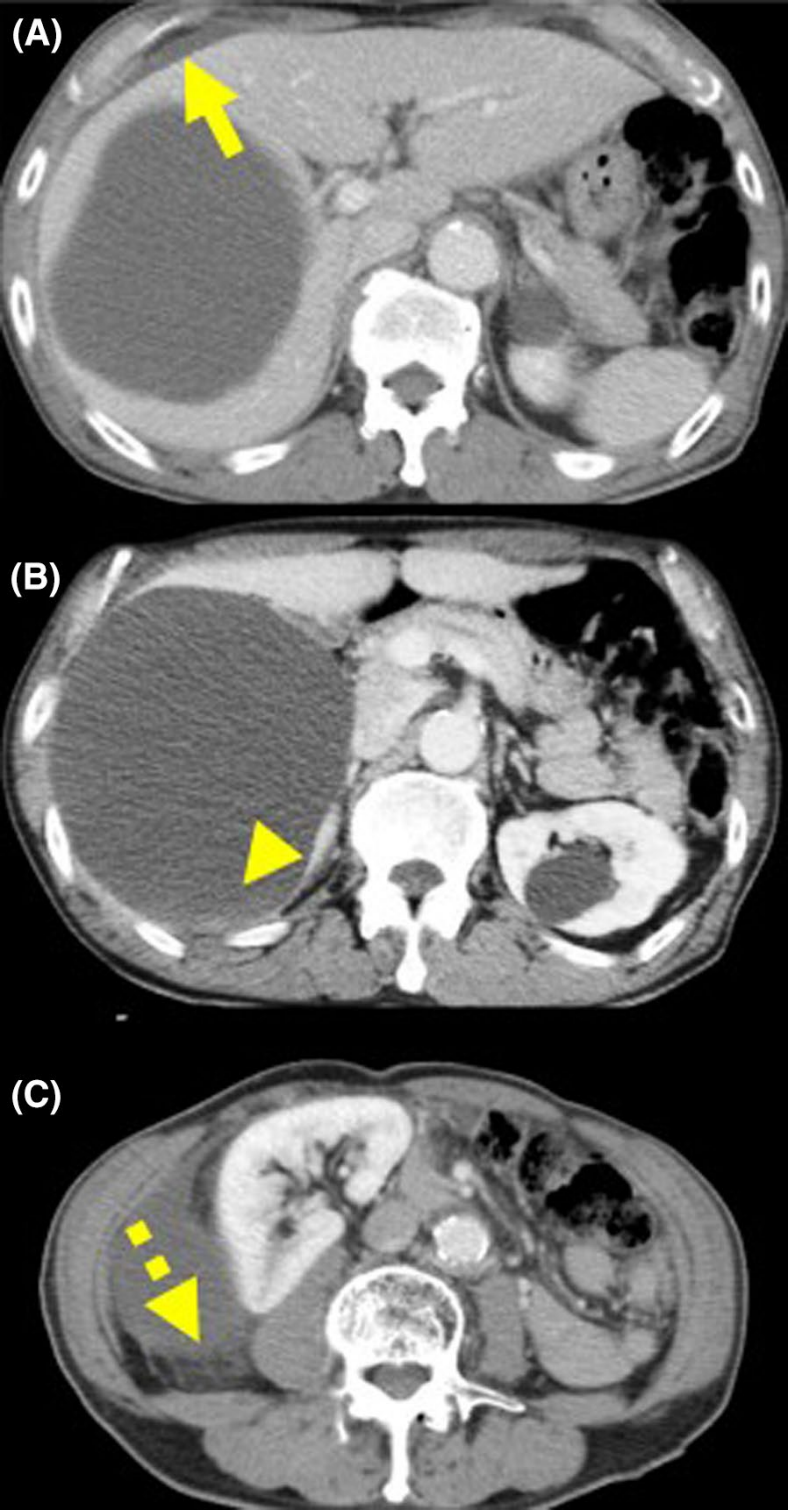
Enhanced CT of case 1. A CT demonstrated a simple hepatic cyst occupying the right lobe of the liver, and fluid collection on the surface of the liver (arrow). B The Hounsfield Unit level was 40 at the lower level of the cyst (arrowhead). C A part of the cystic wall was not smooth but serrated (dotted arrow)
